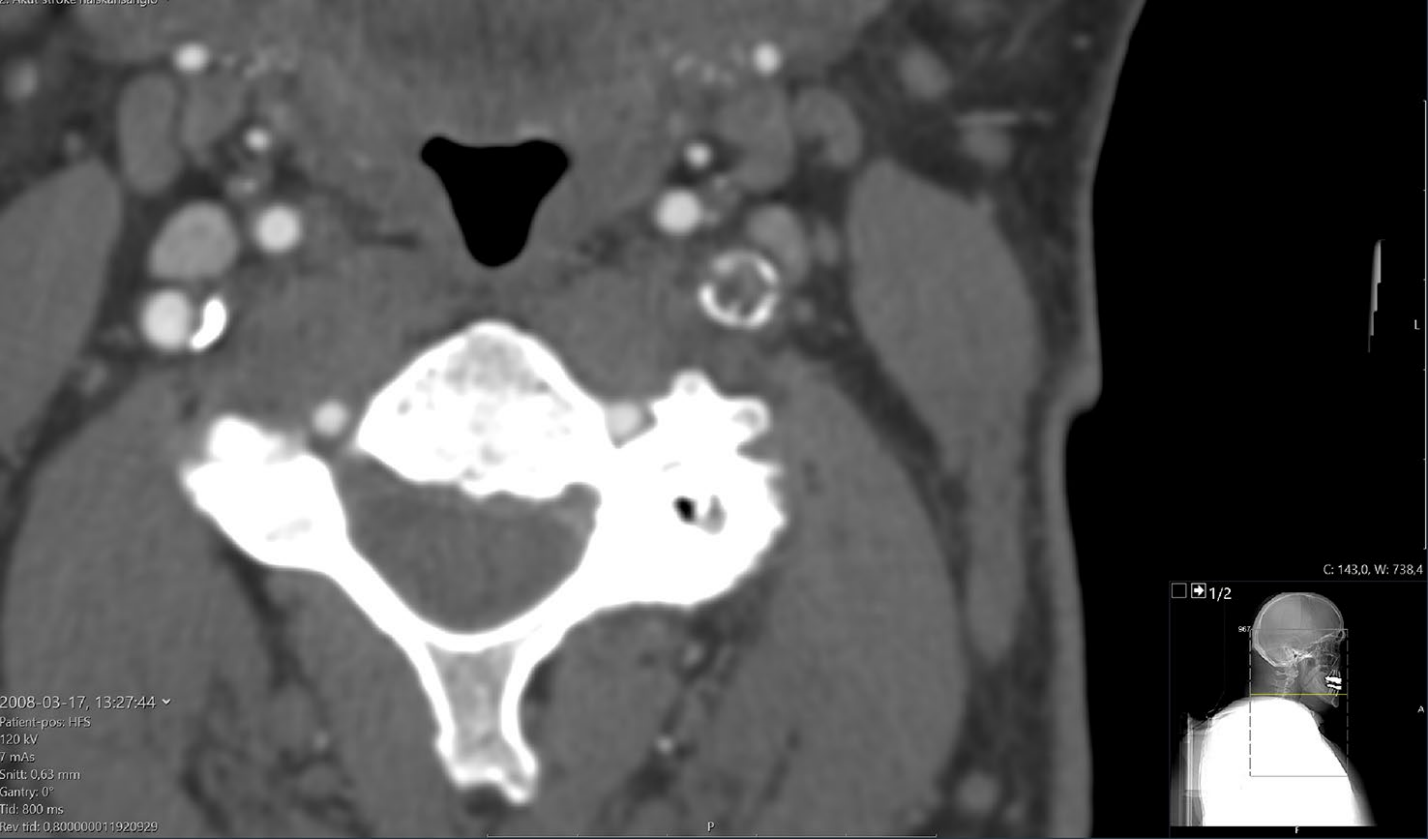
Selective computed tomography-angiography (CT-A) image slice acquired through the clinical examination of patient 1. The location of the image slice is indicted by the inset at the bottom right, and patient characteristics are listed in Table 3
